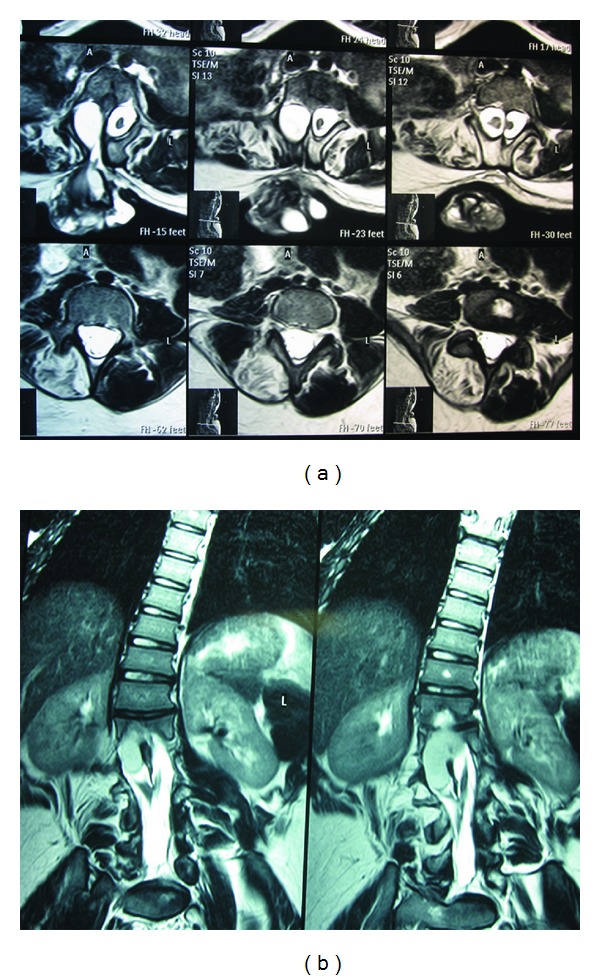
(a) Axial lumbar MRI T-2 images demonstrating a type 1 split cord malformation with the myelomeningocele attached to the right hemicord. (b) Coronal lumbar MRI T-2 images demonstrating a type 1 split cord malformation with a neurenteric cyst attached to the right hemicord.
